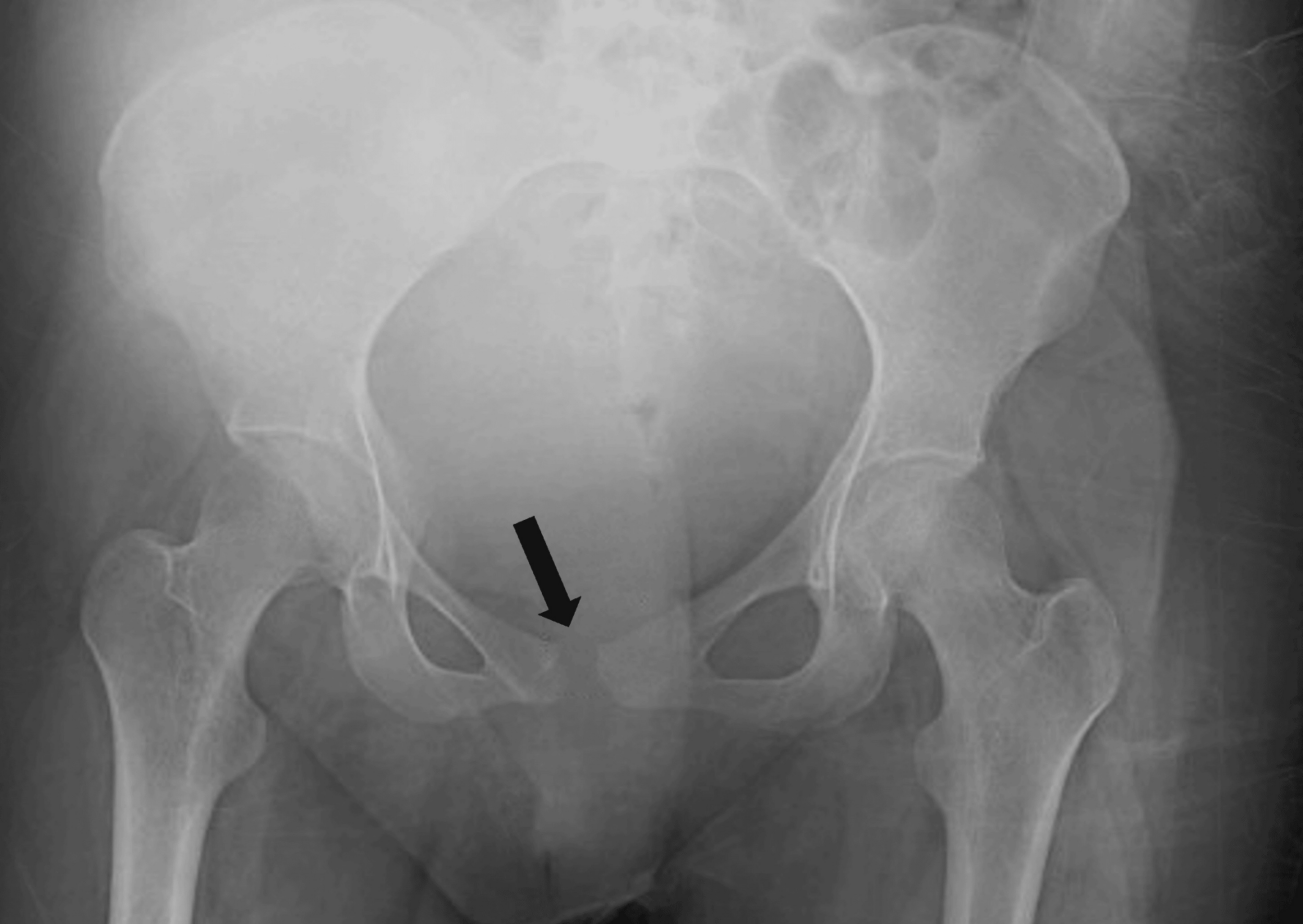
AP pelvis radiograph with black arrow indicating a pubic symphysis diastasis of 1.04 cm Anteroposterior: AP
